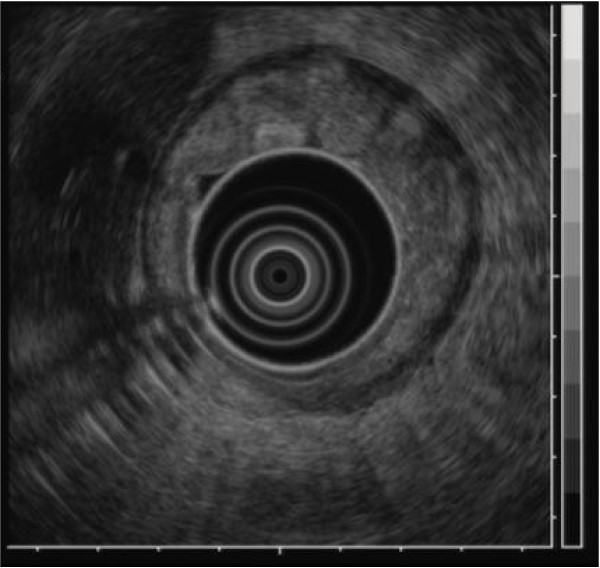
Endoscopic ultrasound findings. Endoscopic ultrasound showing obvious thickening of the submucosal layer without disruption of the gastric wall structure.
